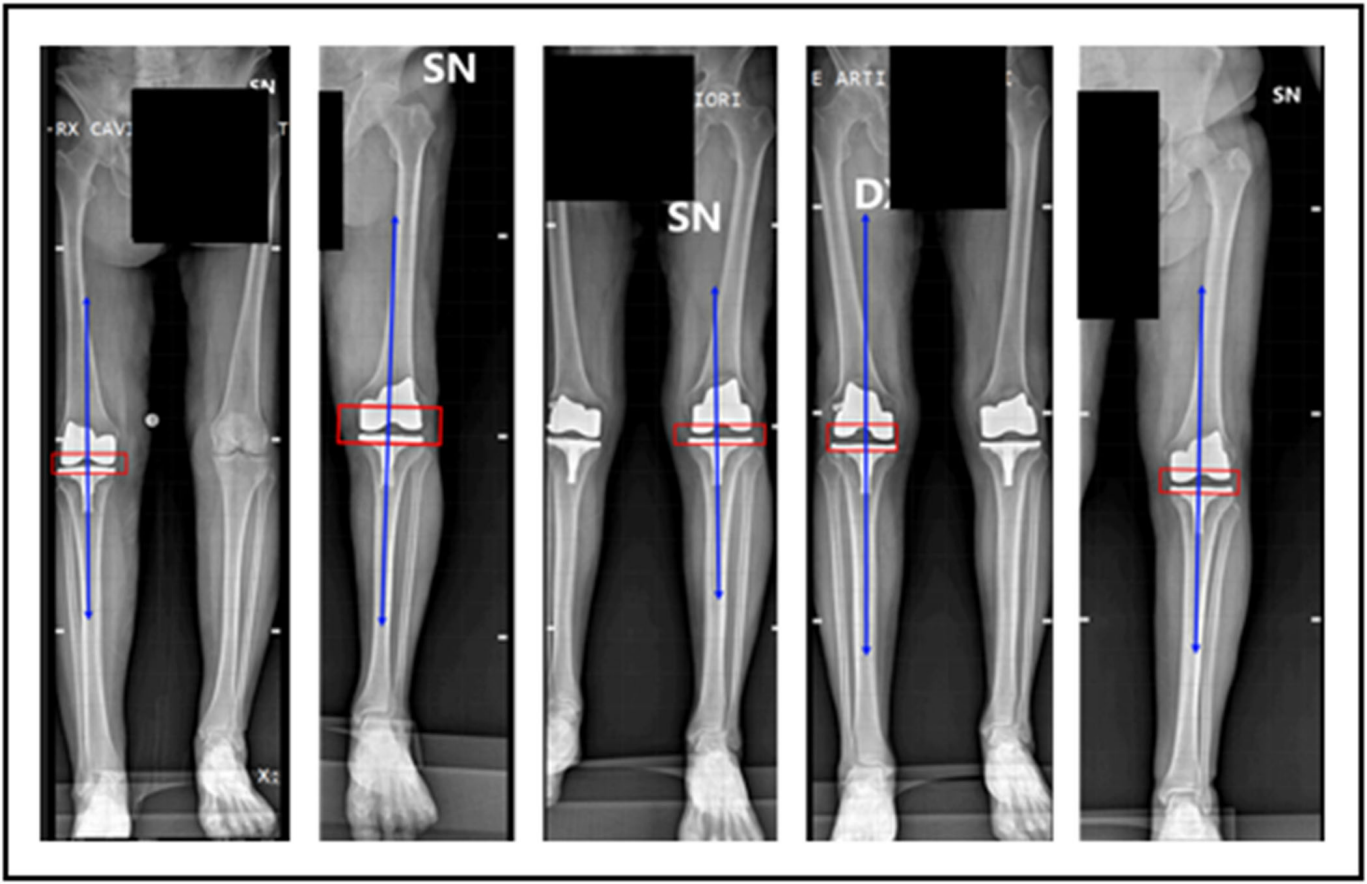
Post‐op radiographs for the validation of physical joint line being parallel to the ground (Symmetric *Smith & Nephew Legion*, Asymmetric *Journey 2*).
